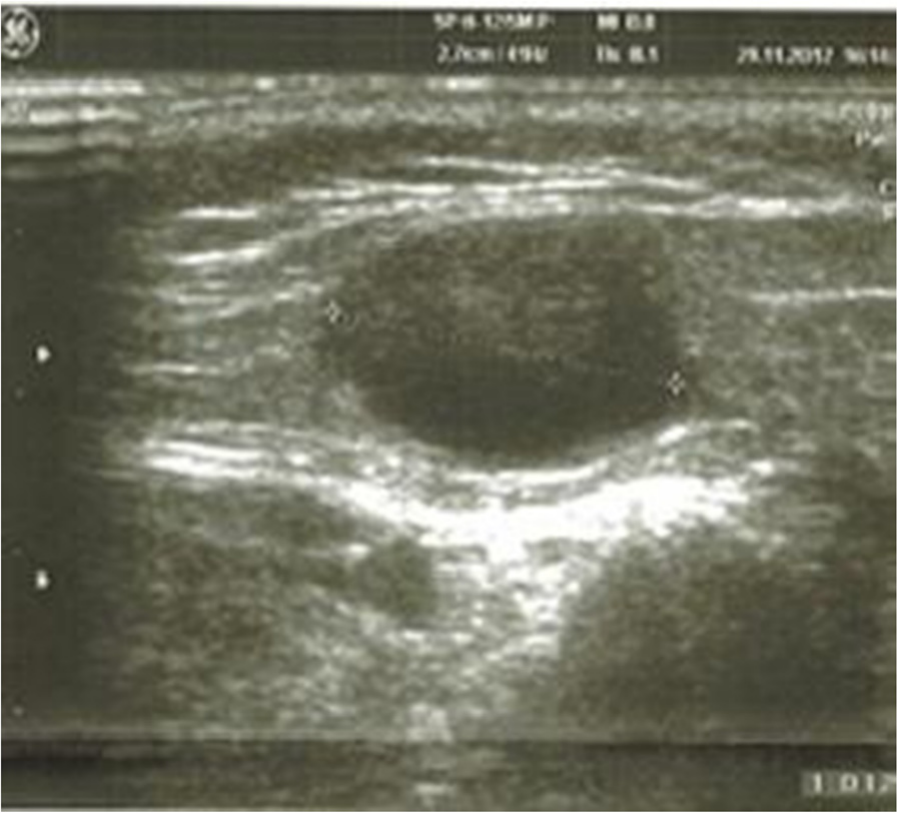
The ultrasound picture of hypoechoic lymph node in the 5-years-old child with CSD
